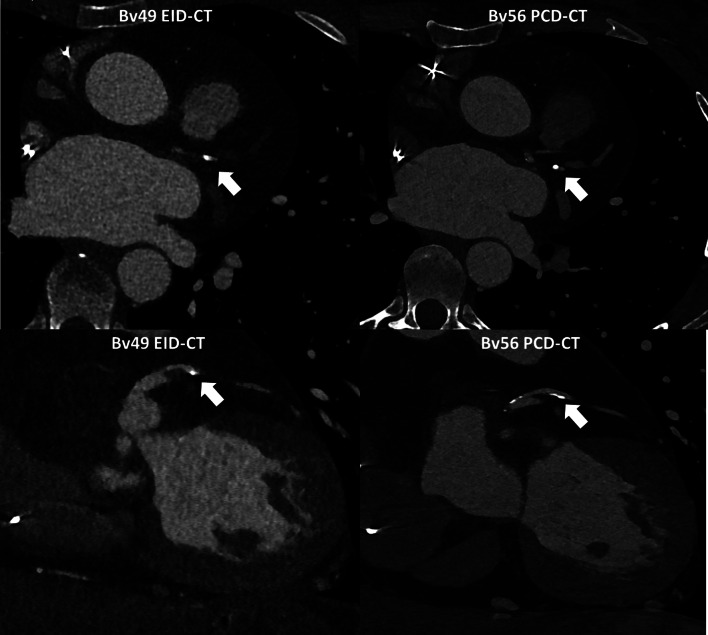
Assessment of coronary stenosis in a 31-year-old heart transplant patient using energy-integrating detector CT and photon-counting detector CT. 31-year-old heart transplant patient with a 50–69% stenosis observed in the obtuse marginal artery on the energy-integrating detector CT (0.6 mm slice thickness, Bv49 kernel). On photon-counting detector CT (0.2 mm slice thickness, Bv56 kernel), the stenosis was downgraded to 25–49%, indicating it was not significant. This example demonstrates that photon-counting detector CT provides enhanced diagnostic confidence compared to energy-integrating detector CT
